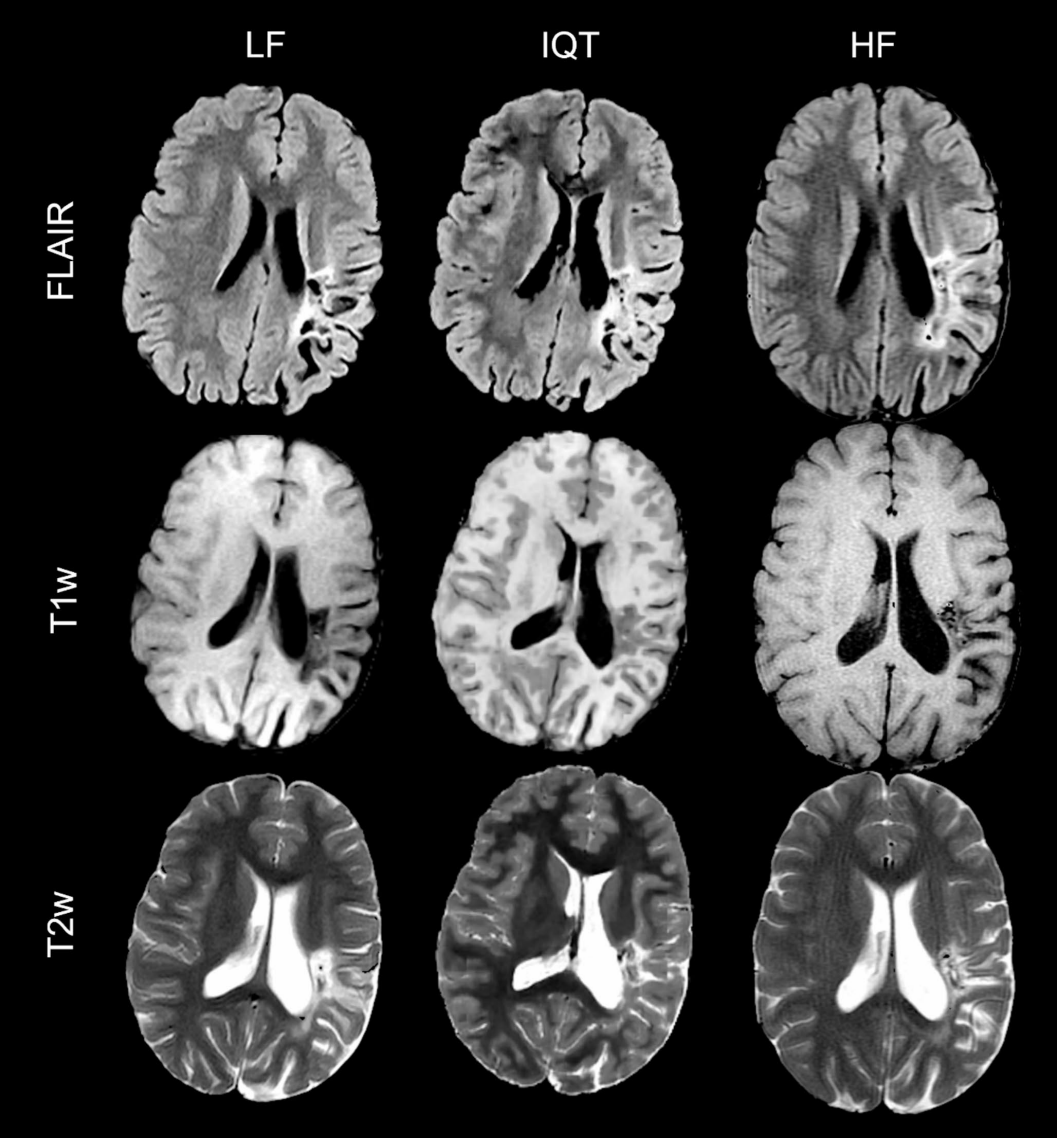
LF, IQT-enhanced and HF images from a 15-year-old patient with long-standing encephalomalacic damage in the middle cerebral artery territory on the left from previous perinatal ischemia. The malacic area is better seen on T2w and FLAIR images, while the partial voluming in IQT on T1w images makes the brain cortex look thick. In this case, the 3D visualisation allowed by IQT, even though advantageous compared to the original 2D images, is not completely accurate and may have negative consequences on lesion identification, which stresses the importance of having multiple sequences
